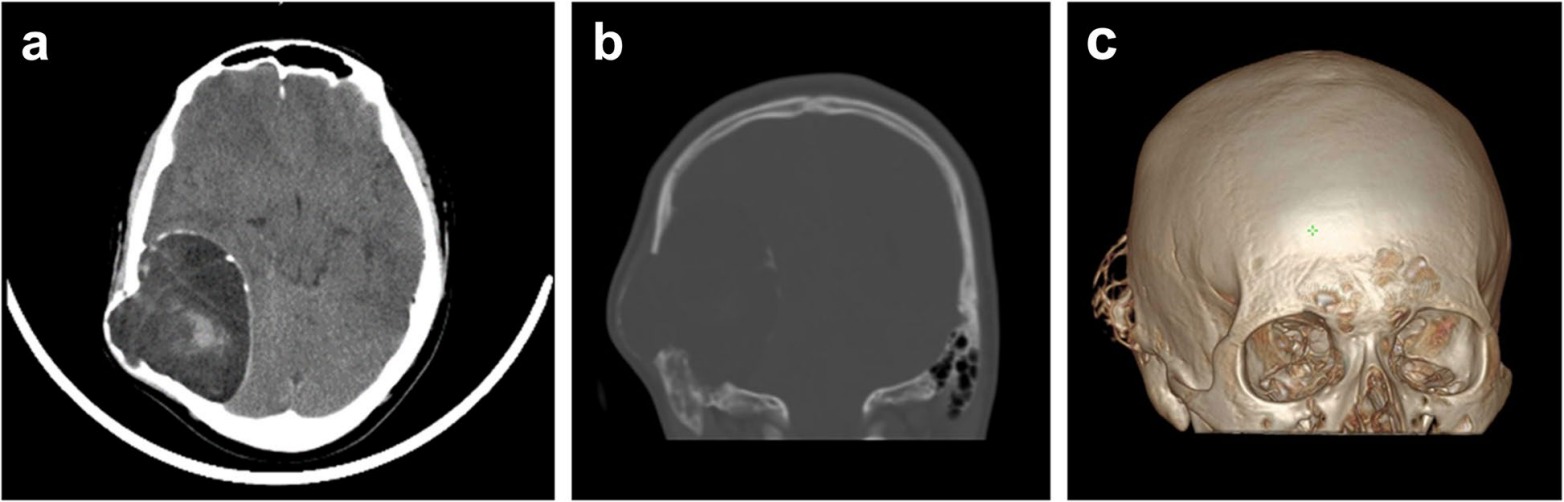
Head computed tomography (CT) (a-c) scan shows a right temporal mass with cystic and solid components (a, axial, soft tissue window). The density of the mass is mostly the same as that of liquid (a). Right temporal bone is obviously destroyed (b, coronal, bone window) (c, three-dimensional reconstruction)
